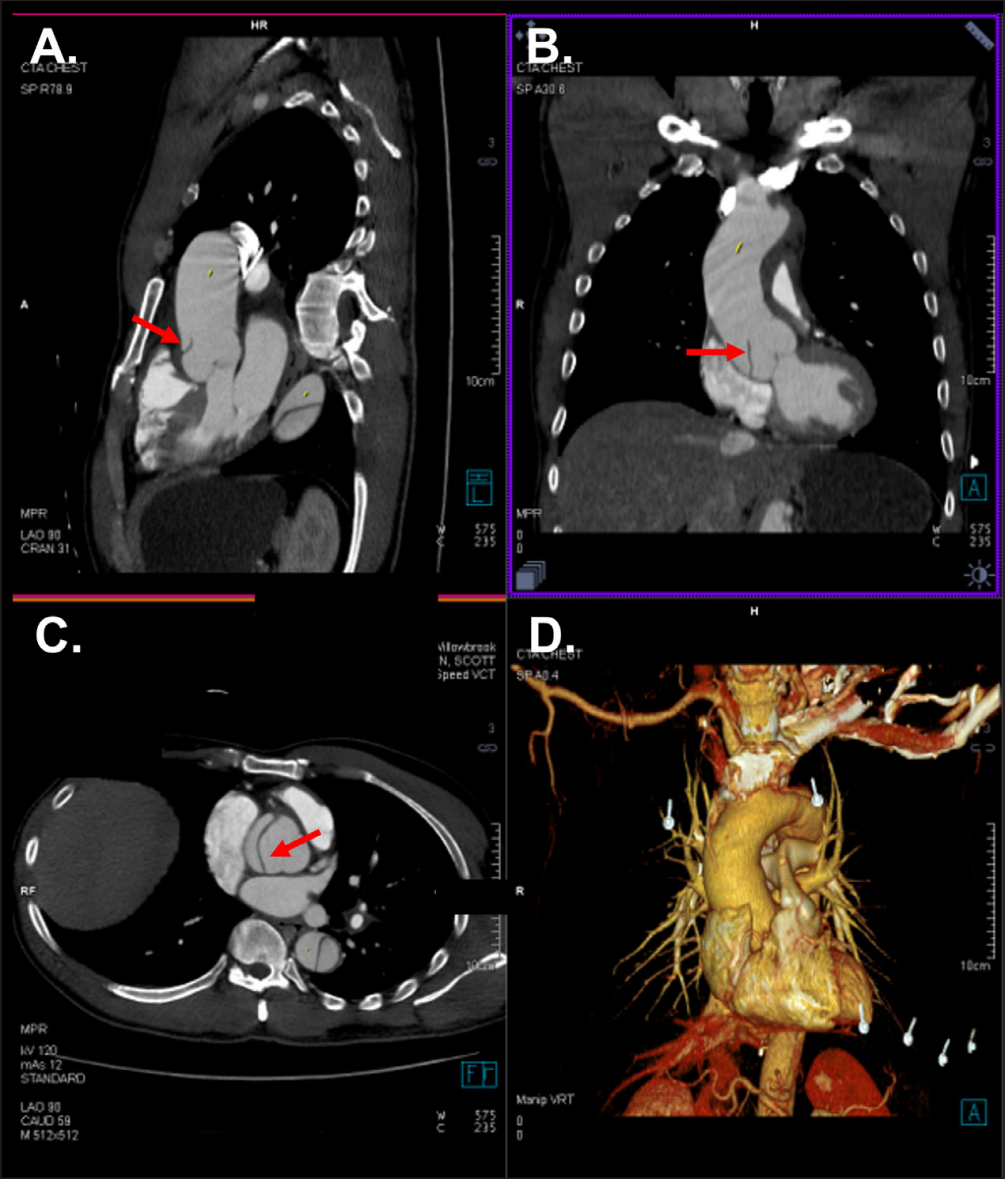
Aortic root dissection. Sagittal (A), coronal (B), and transverse (C) computed tomography showing dissection flap in the aortic root (arrow). Three-dimensional reconstructed computed tomography is provided (D).
